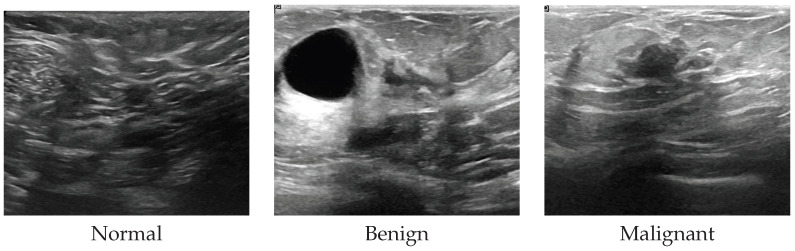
Representative breast ultrasound images showing normal tissue, benign lesions and malignant tumors.
